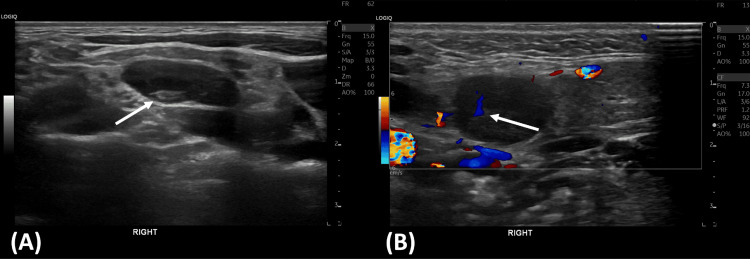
(A) Ultrasonographic imaging of the right cervical lymph node with maintained hilum (arrow). (B) Doppler ultrasonography showing a right cervical lymph node with maintained vascularity (arrow).
